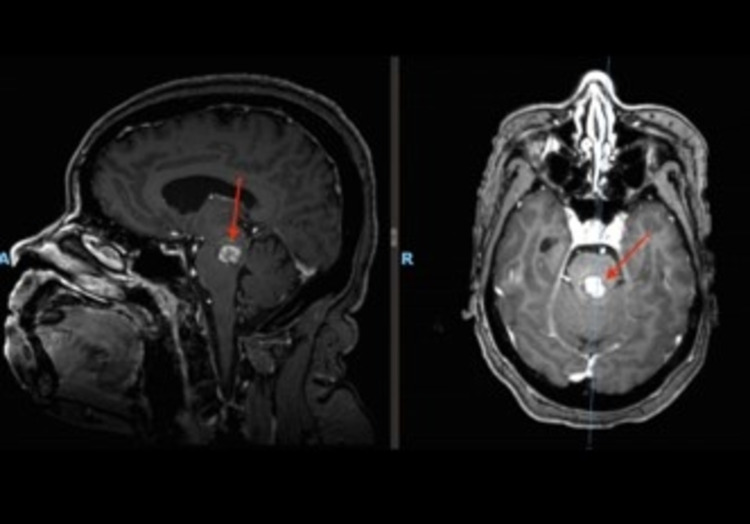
Case 1: axial and sagittal MRI revealing an intra-axial midbrain cavernoma
